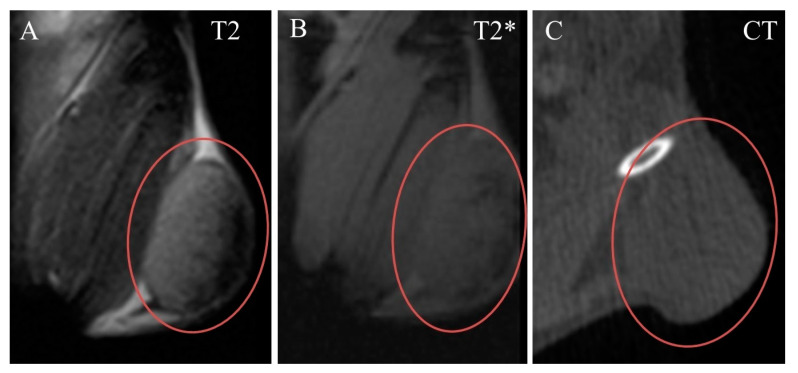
Native coronal images of Ca755 mammary carcinoma. (A) T2 MRI; (B) T2* MRI; (C) CT image. The tumor is located on the right hind leg.
